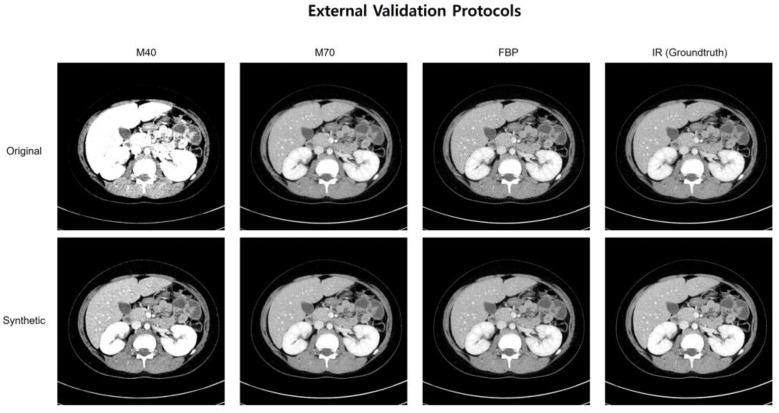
A representative case of abdominal CT before and after harmonization in the external validation set.
